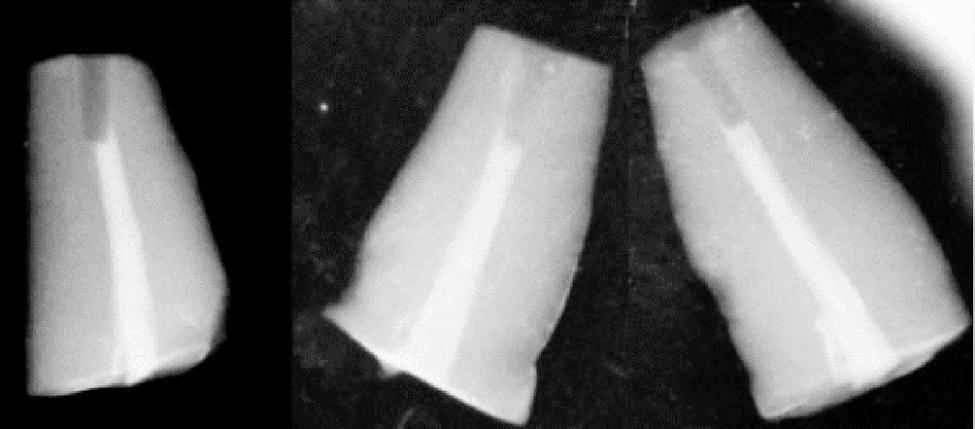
Radiographic view of the roots after cutting the apical 3 mm and creating a cavity with 3 mm depth (by gutta-percha removal) at the root end using an ultrasonic scaler
